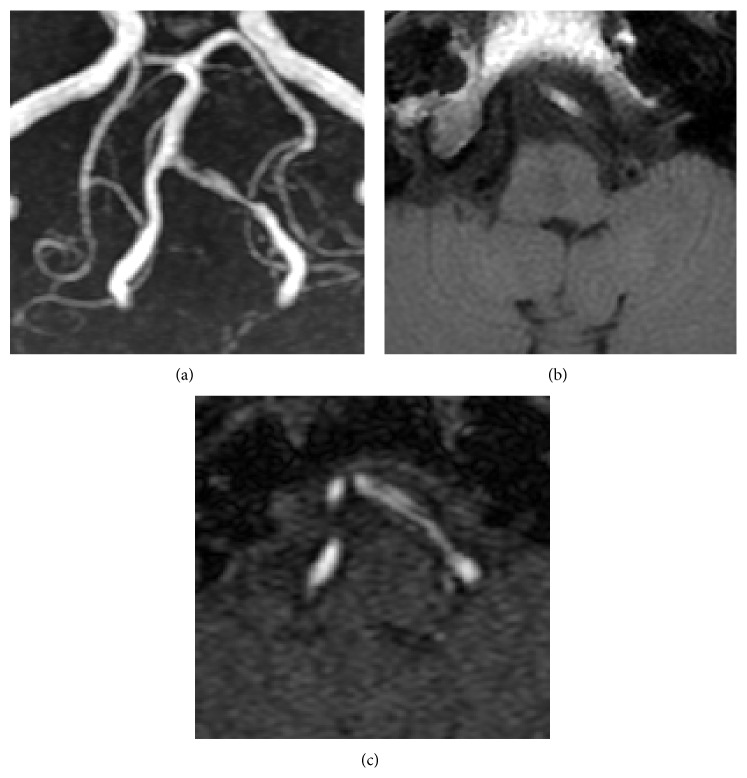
(a) Time-of-flight magnetic resonance angiography (MRA) of the left vertebral artery on admission reveals irregular narrowing of the lumen and a surrounding high-intensity area suggestive of a false lumen or intramural hematoma. (b) The T1-weighted image of MRI demonstrates a high-intensity area in the vessel wall, indicating intramural hematoma. (c) The source image of the MRA demonstrates a linear low-intensity structure presumed to be the intimal flap that separates 2 high-intensity areas.
